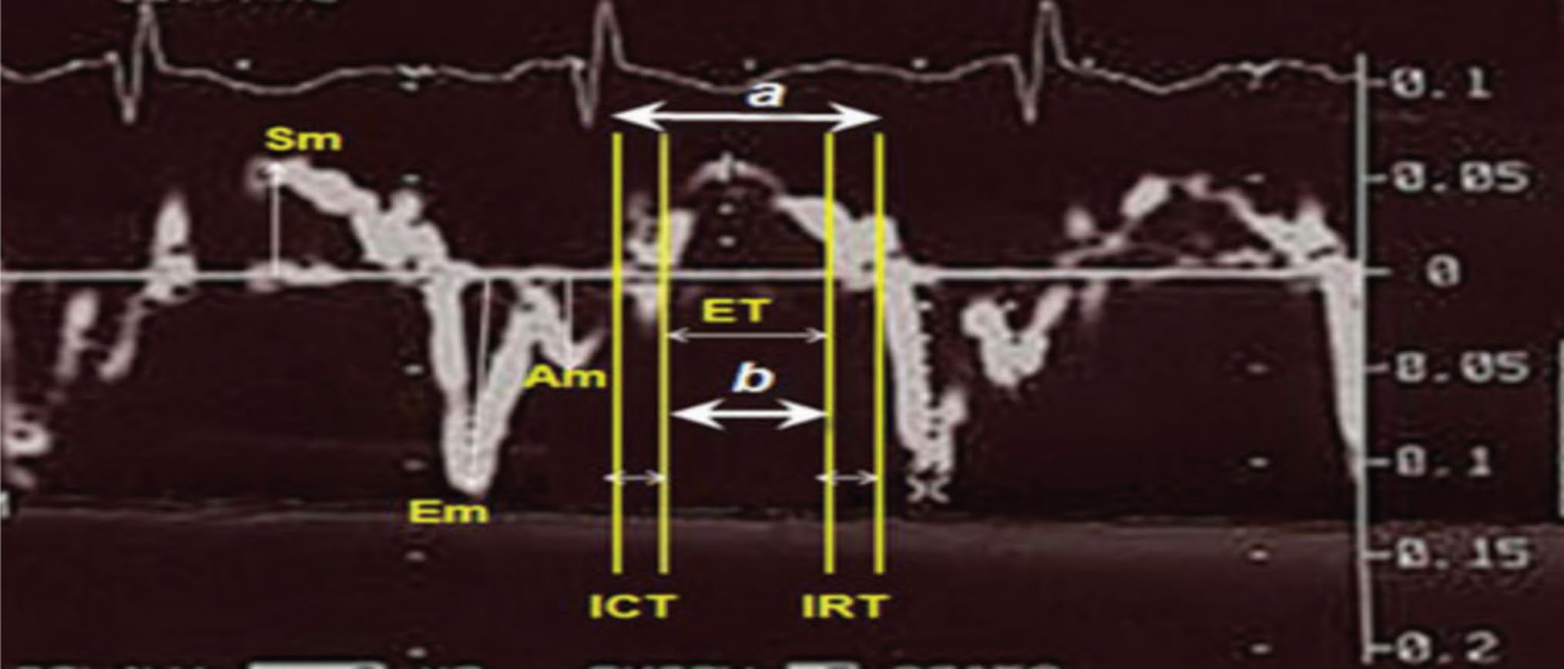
Example of calculation of left ventricular Doppler tissue imaging (DTI) measurements
